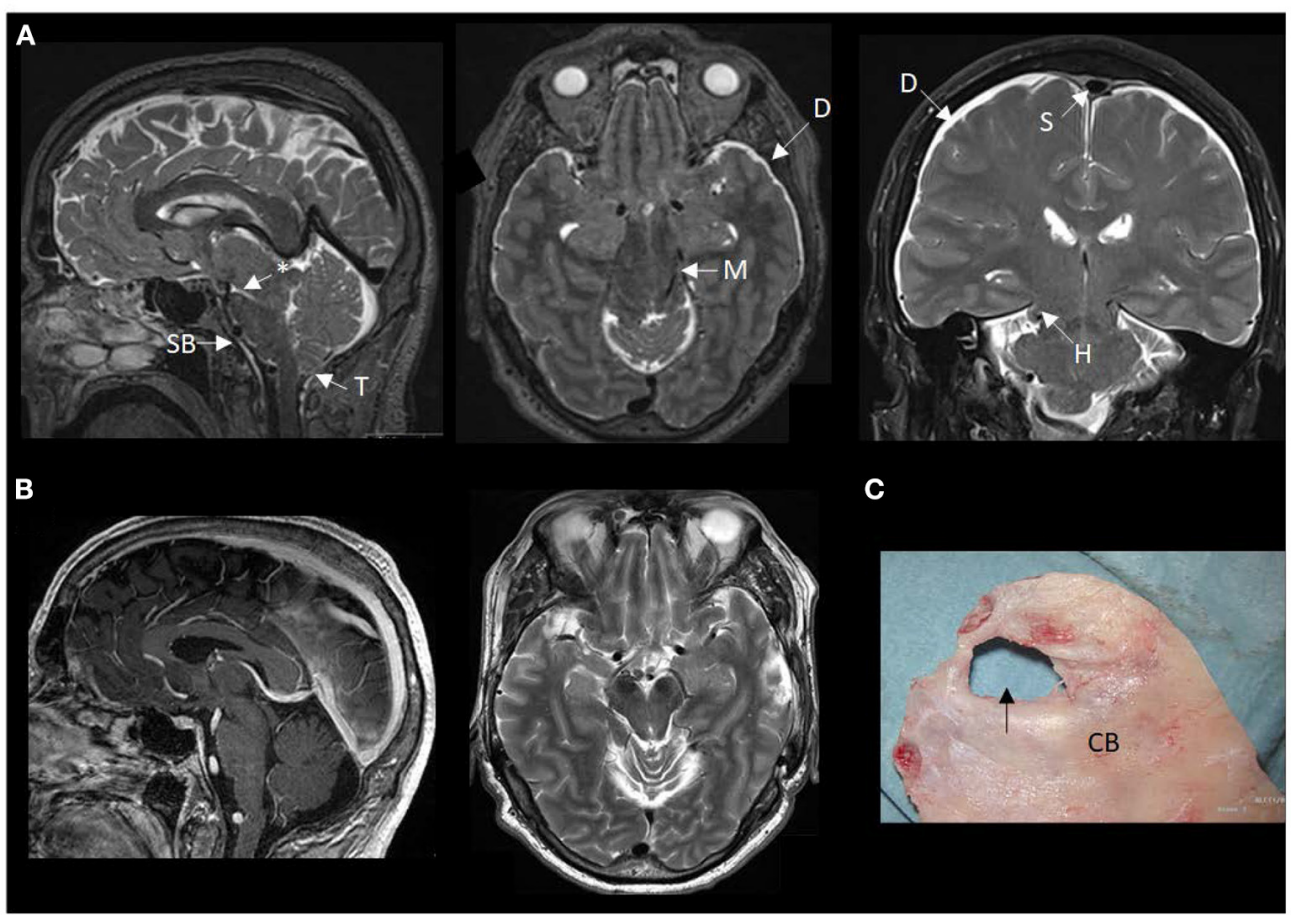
MRI findings of brain sagging dementia in this case. (A) The preoperative MRI in sagittal, axial and coronal planes show typical signs of intracranial hypotension, including sagging brainstem (SB) toward the clival bone, downward tonsillar (T) ectopy, smaller ponto-mesencephalic angle (indicated by an asterisk), enhancement of the dura (D), rounding of the cross-section of the dural venous sinus (S) indicative of dural venous engorgement, transtentorial herniation (H), and reduced space for the mesencephalon (M). (B) The postoperative MRI in sagittal and axial planes demonstrate reversal of the MRI signs of brain sagging. (C) The cranial bone (CB) with a defect (indicated by an arrow), as presented during surgical exploration.
